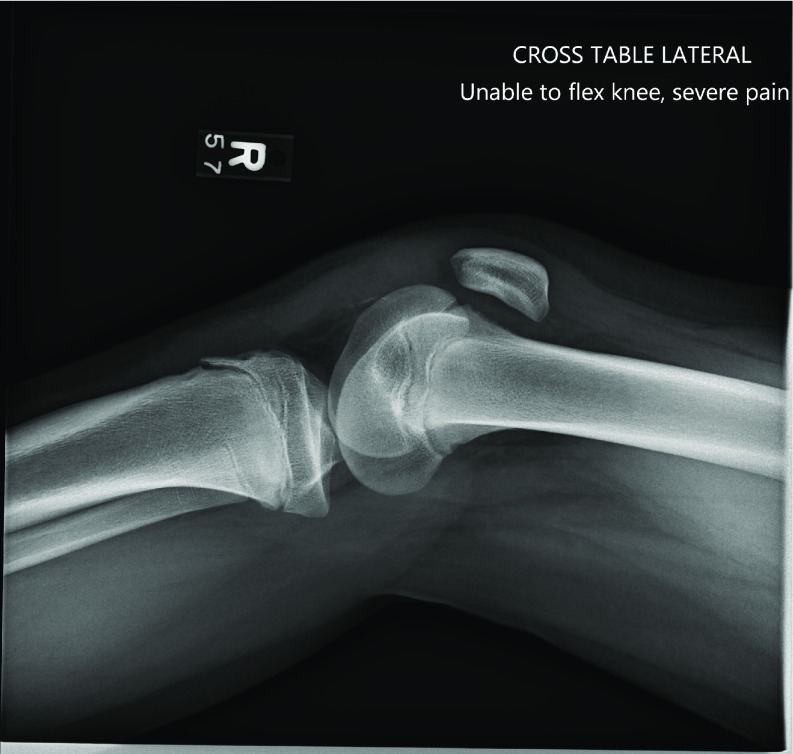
Lateral preoperative radiographs of the right knee for the patient described in Case 1. Patella alta is evident with possible patella sleeve fracture and accompanying avulsion of the tibial tubercle apophysis.
